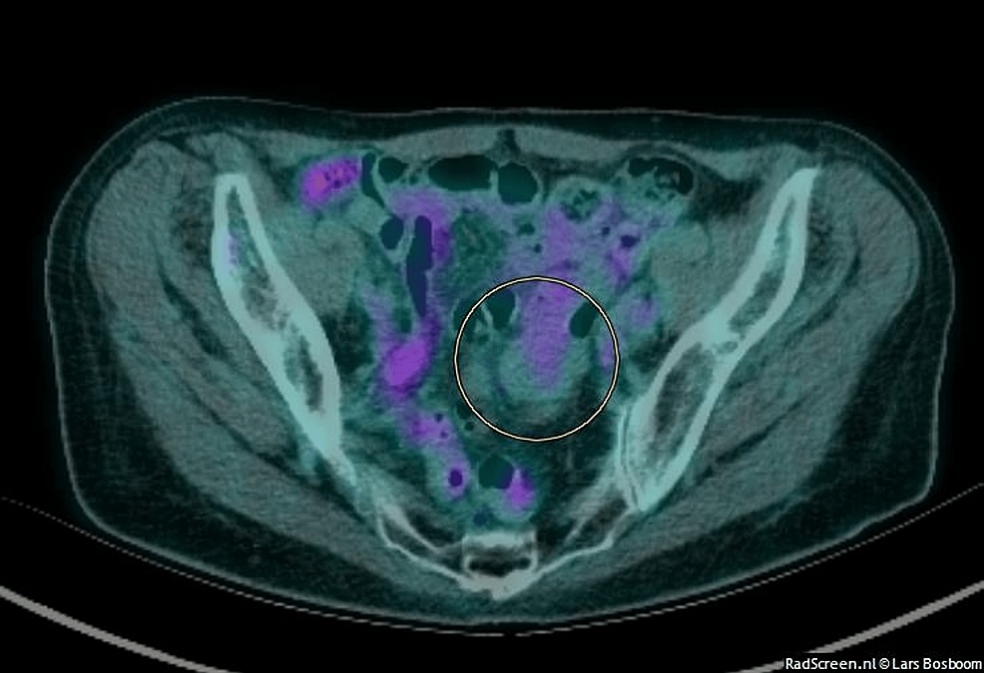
Axial fused 18F-FDG PET/CT image The circle highlights the background level uptake in the solid mesenteric mass. 18F-FDG PET/CT: Positron emission tomography (PET) with 2-deoxy-2-(fluorine-18) fluoro-D-glucose integrated with computed tomography
